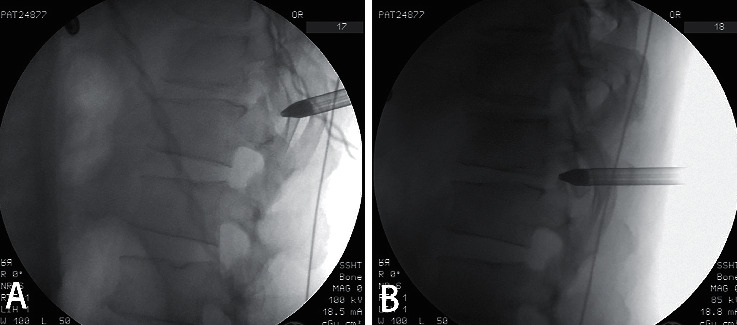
Images of X-ray fluoroscopy showing the dilator punching procedure: a dilator with blunt tip located on the lateral side of the articular process (a). The dilator slid into the lower part of the intervertebral foramen (b).
